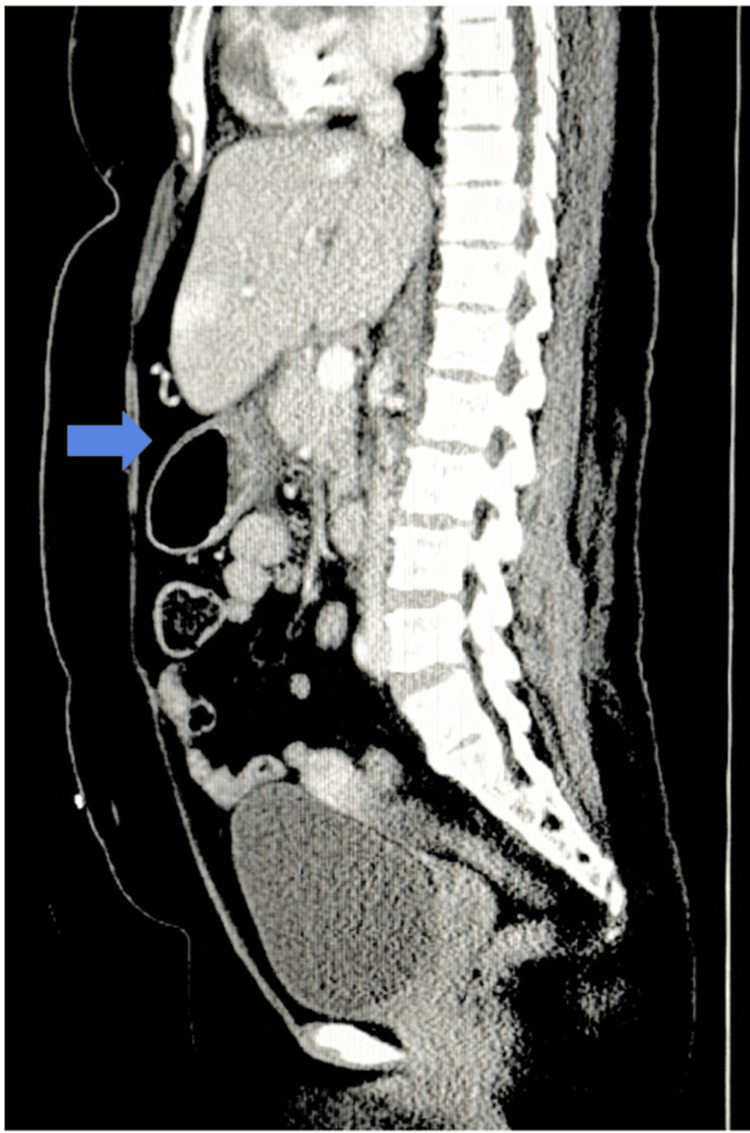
Sagittal CT reconstruction demonstrating peripancreatic inflammation adjacent to the stomach. Sagittal reconstruction demonstrating peripancreatic inflammatory fluid tracking adjacent to the greater curvature of the stomach, with associated gastric wall thickening (arrow, reflecting reactive inflammatory changes secondary to pancreatitis). CT: computed tomography
